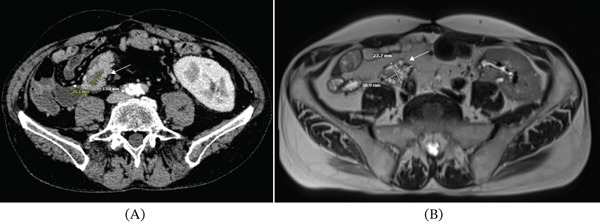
Follow‐up of the cystic pancreatic lesion using (A) contrast‐enhanced CT in March 2024 and (B) T2‐weighted MRI in November 2024, showing a substantial increase in size within a short period of time.
